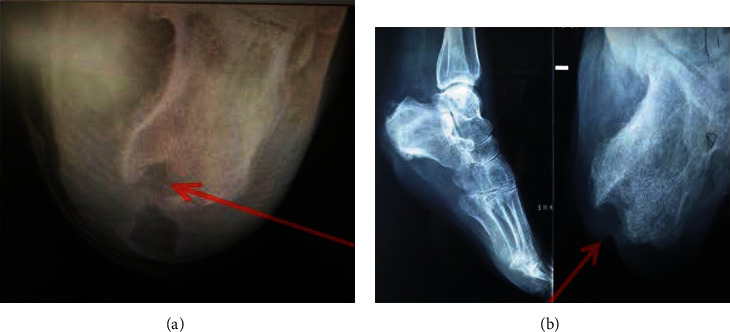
Clinical radiographies of diabetic heel ulceration (a) before surgery and (b) 12 weeks after surgery. The arrow head is pointed on the calcaneus.
